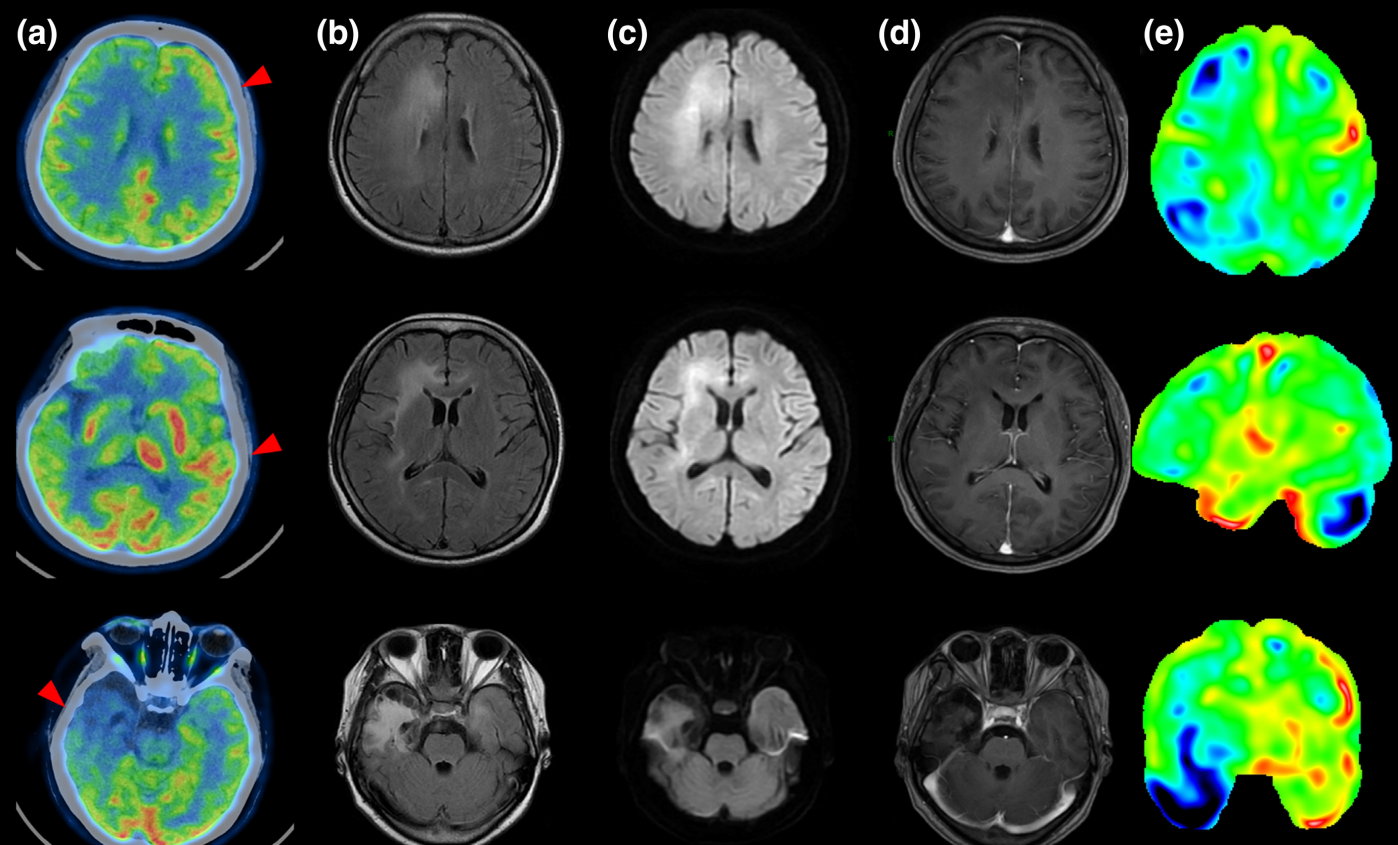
A typical case of the PET scan time is 2–3 months from symptom onset (pat. 17 had intermittent fever for 2 months, cognitive impairment, and speech disorders accompanied by abnormal behavior). FDG‐PET (A) at approximately 63 days revealed that hypermetabolism in left super temporal, hypometabolism in the left middle frontal and right frontal‐ parietal‐temporal lobes (especially in the right temporal lobe), which correspond to mild hyperintense in MRI areas. T2‐ FLAIR (B) and DWI (C) of axial MRI in 49 days showed multiple asymmetric white matter hyperintensities, especially in the right frontal‐temporal lobe, and lateral ventricle. There was a lack of enhancement in mentioned above areas of contrast‐enhanced MRI (D). The FDG statistical deviation images analysis based on the *z*‐score (E).
